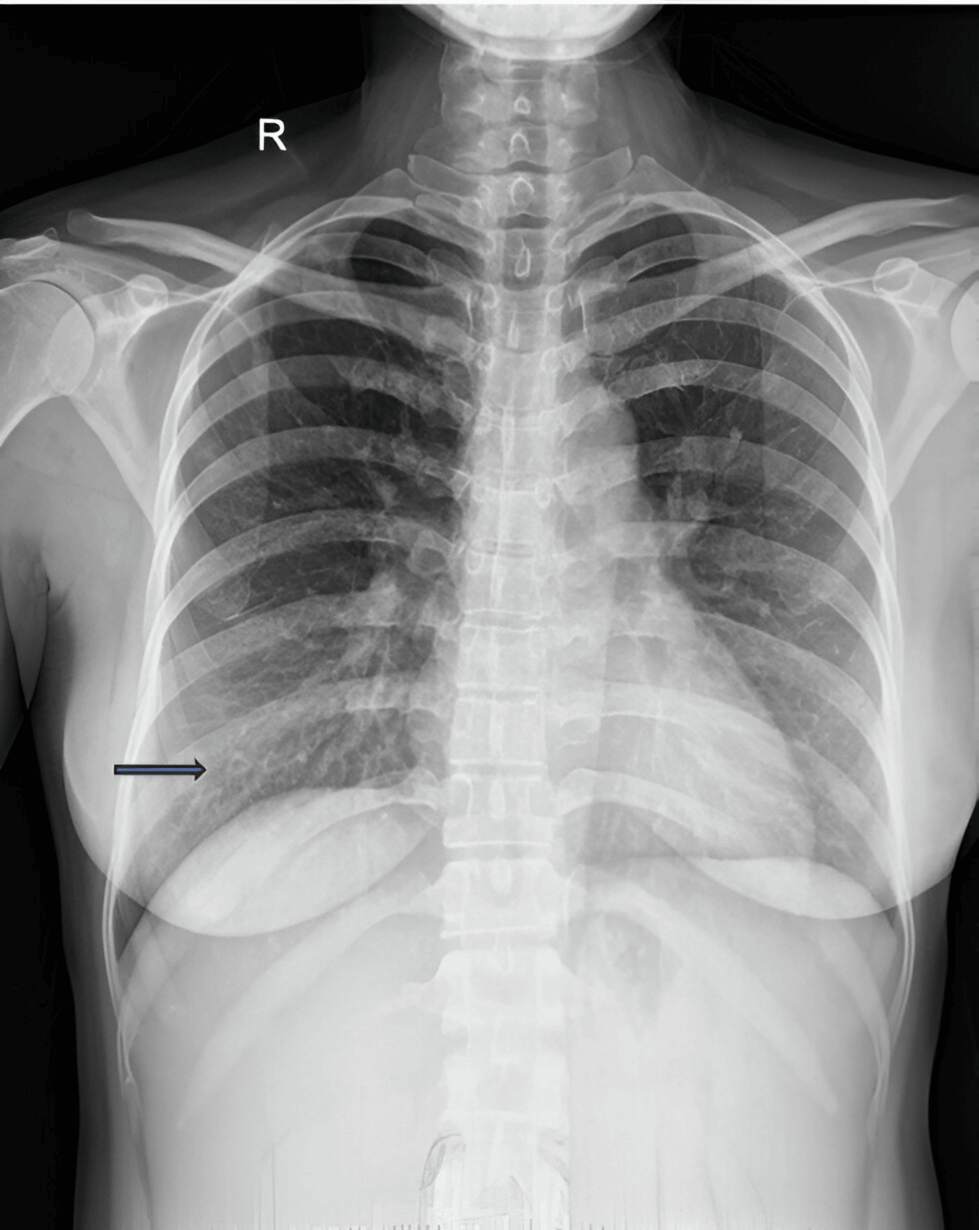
Chest X-ray upon admission The arrow points to small shape cavities suspicious of micro-abcesseses.
